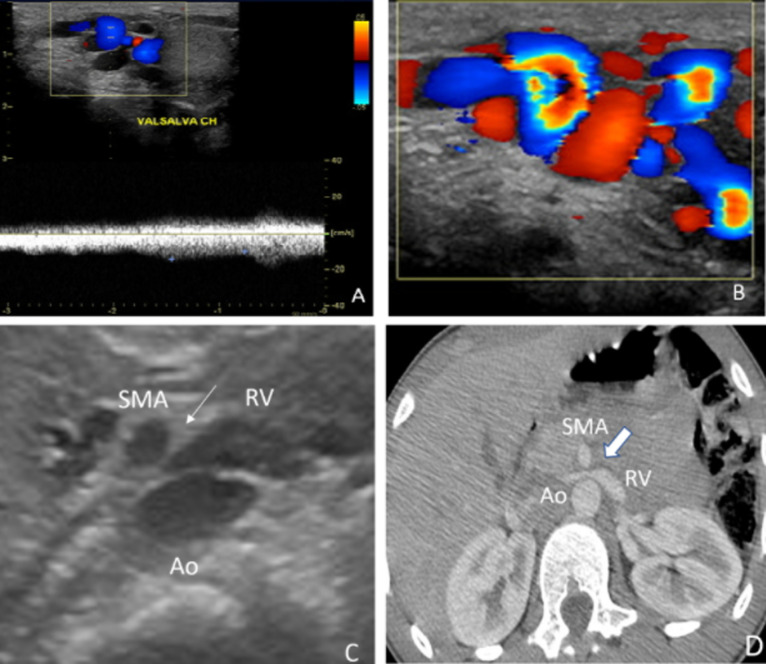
Doppler ultrasound (US) of the left pampiniform plexus and the renal vessels shows varicocele grade 3, as a dilatation of the left pampiniform plexus of veins with reversal flow during the Valsalva maneuver (A, B) and reveals an associated nutcracker phenomenon (C, arrows); computed tomography (CT) scan with contrast shows also the compression of the left renal vein (RV) between the superior mesenteric artery (SMA), and the abdominal aorta (AO) confirming the nutcracker phenomenon (NCS) (D, arrows)
